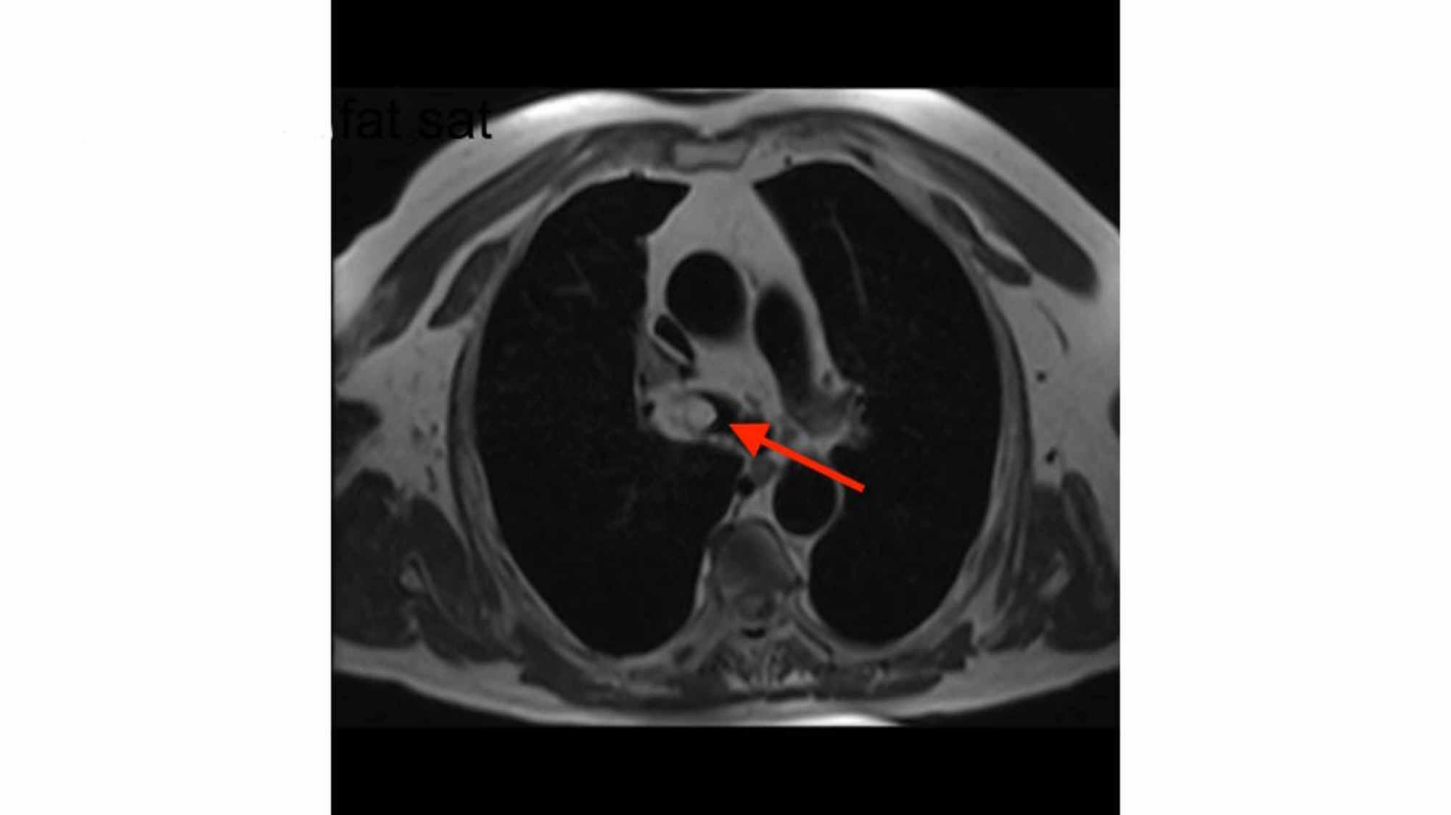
T2-weighted magnetic resonance imaging (MRI) shows fat density obstructive lesions in the right bronchus
